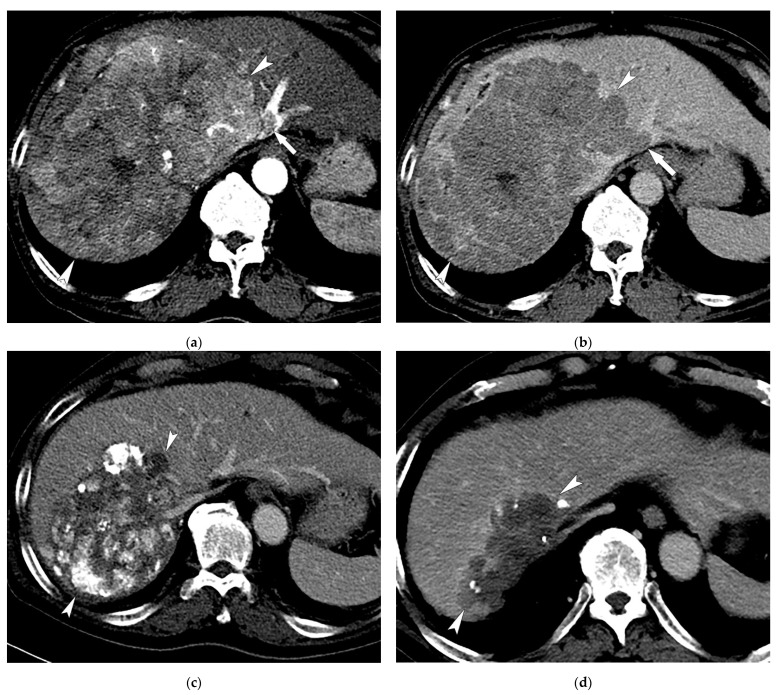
Images of a 59-year-old male patient with multinodular HCCs with MVI who received TACE combined with sorafenib treatment. Contrast-enhanced axial CT images in the arterial (a) and delayed (b) phases show a huge enhancing mass (17 cm in maximal diameter; arrowheads) invading the left hepatic vein (arrows). (c) CT image at 1 year after 4 sessions of TACE combined with sorafenib therapy shows necrotic change with lipiodol uptake in the tumor and a decrease in tumor size (13 cm; arrowheads). (d) CT image at 3 years after 6 sessions of TACE shows a further decrease in tumor size without tumor viability (9 cm; arrowheads).
